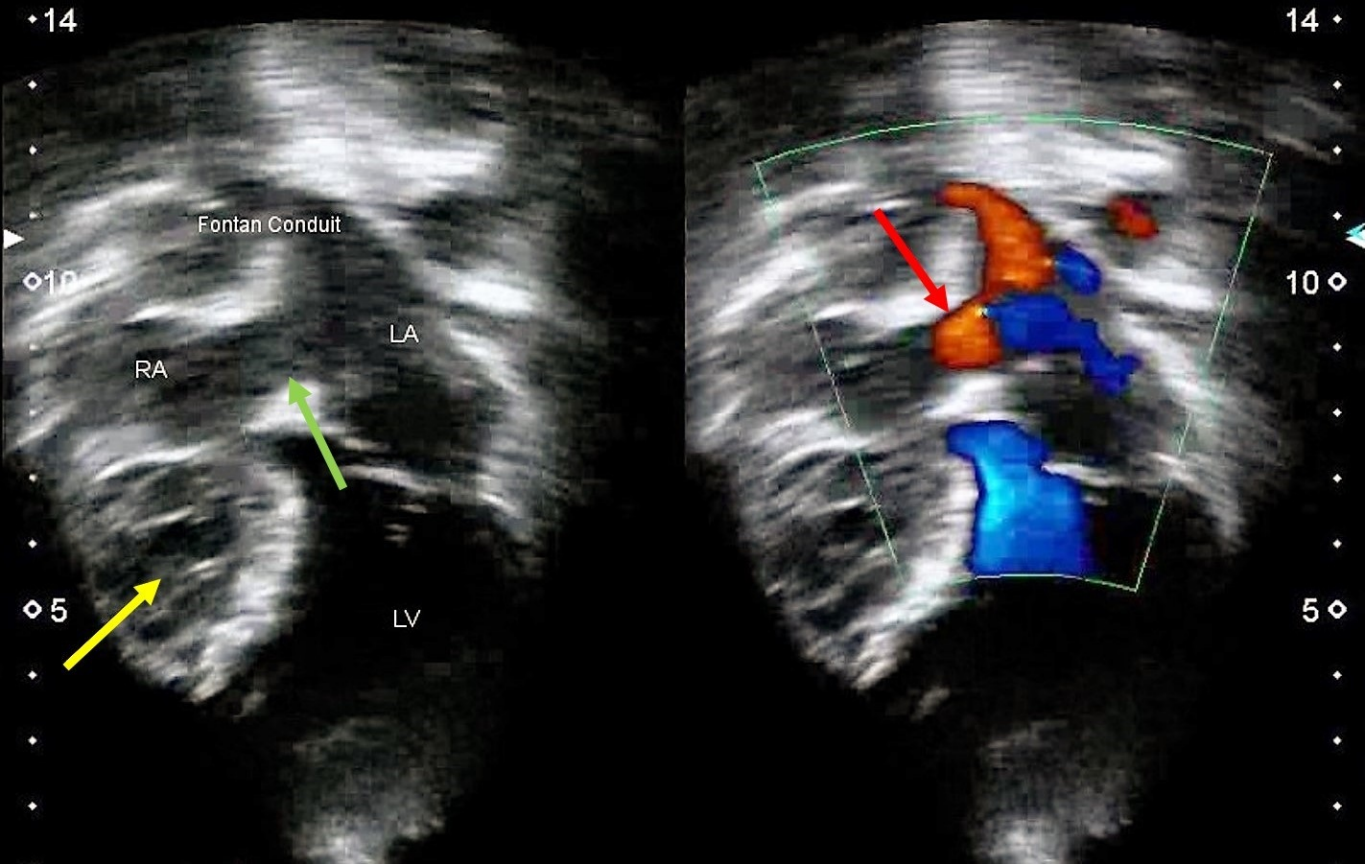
Echocardiogram showing a hypoplastic right ventricle (yellow arrow) and an atrial septal defect (green arrow) with no intracardiac thrombus formation. Color doppler imaging demonstrating blood flowing from the right to the left atrium (red arrow)
